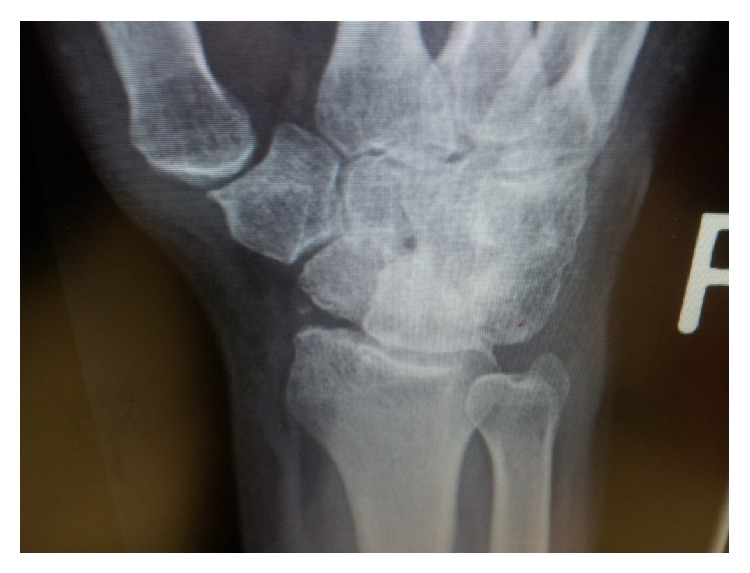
Postdistraction radiograph showing improved radiocarpal appearance.
